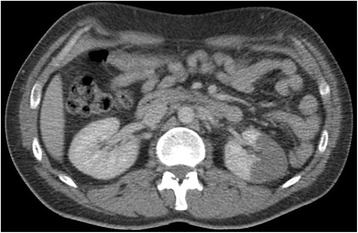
A contrast-enhanced abdominal computed tomography scan performed a day after the first visit reveals a renal infarction of the left kidney
